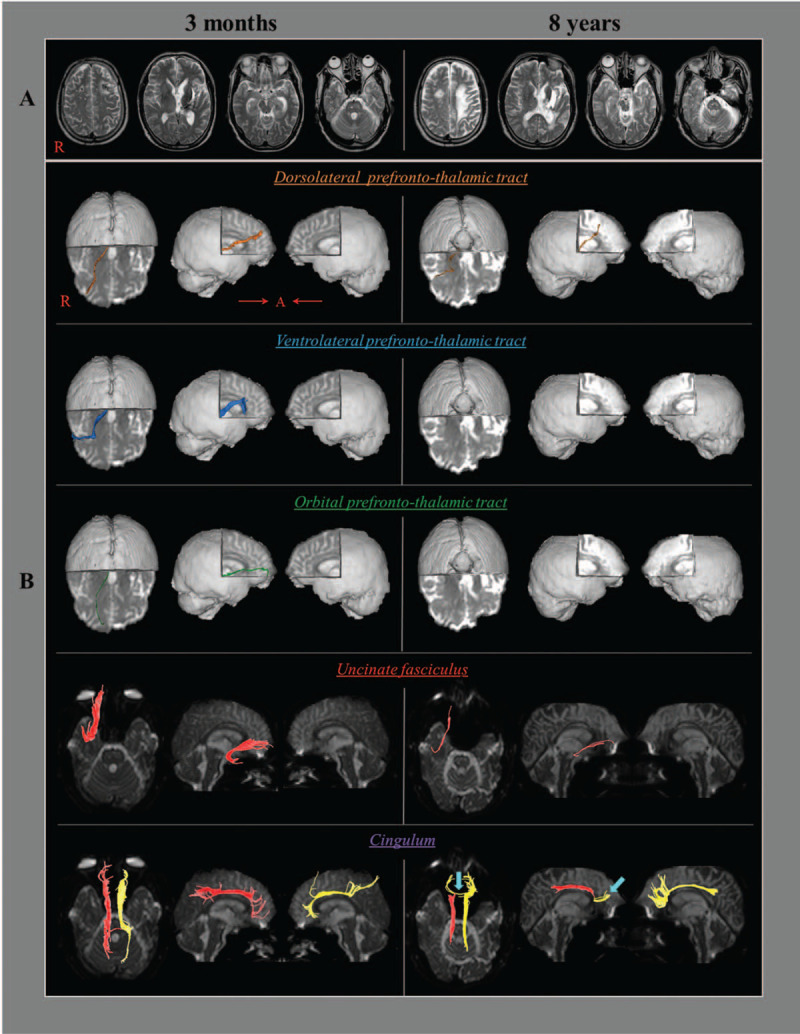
(A) Brain magnetic resonance images obtained at 3 months and 8 years after traumatic brain injury onset show leukomalactic lesions in the left basal ganglia and subcortical white matter. (B) On both 3-month and 8-year diffusion tensor tractographies (DTTs), the right dorsolateral prefronto-thalamic tract (DLPTT), the ventrolateral prefronto-thalamic tract (VLPTT), the orbital prefronto-thalamic tract (OPTT), the uncinate fasciculus, and both cinguli are reconstructed, whereas other neural tracts are not reconstructed. All reconstructed neural tracts on 8-year DTT are narrow compared with the 3-month DTT, except for the left cingulum which shows new transcallosal fibers (arrow) between both anterior cingula.
